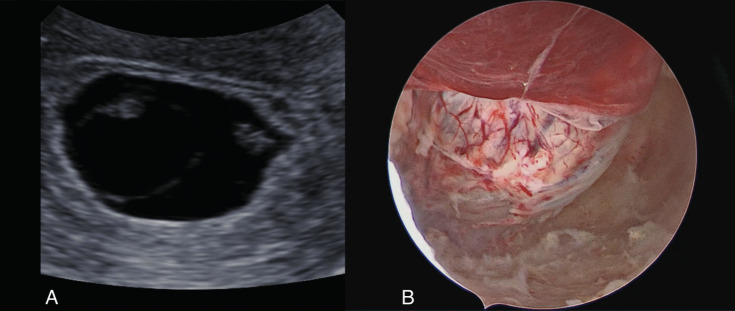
Preoperative images of the missed miscarriage: A) Preoperative ultrasound image; B) Hysteroscopic vision of the GS.
